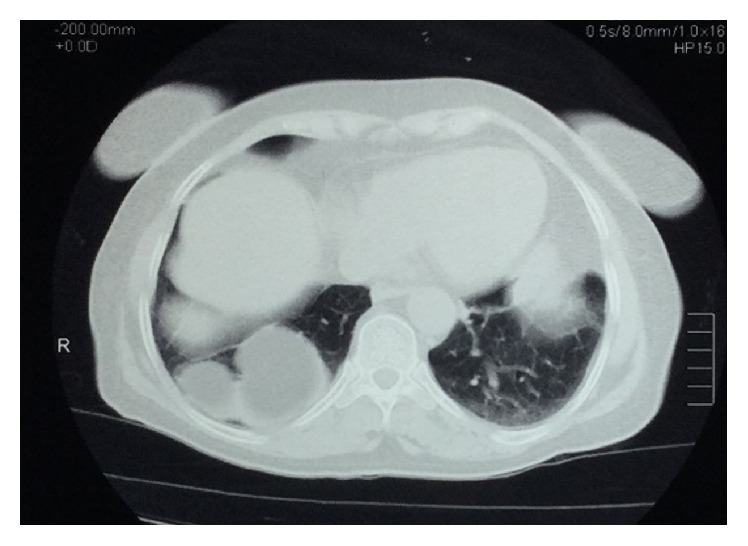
Contrast-enhanced CT scan showed right diaphragmatic hernia.
